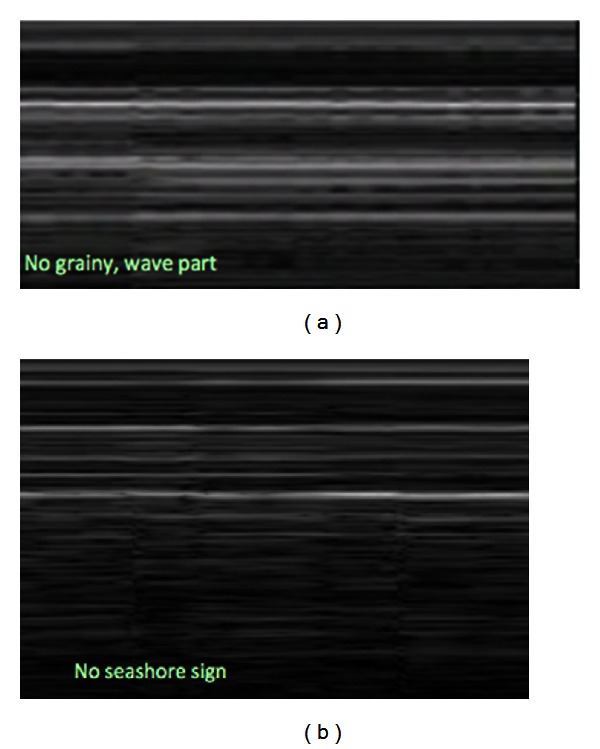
Pneumothorax diagnosed using ultrasound.
